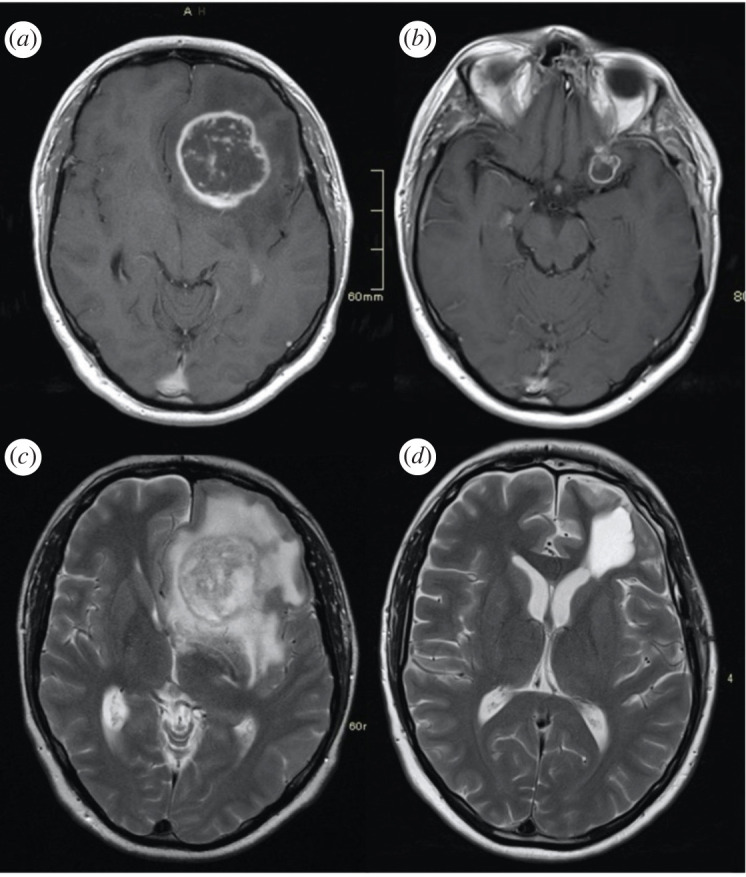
Representative images for a patient with remote recurrence of GBM in the contralateral hemisphere. T1-weighted post-gadolinium images (*a*,*b*) and T2-weighted images (*c*,*d*). (*a*) Pre-operative diagnostic imaging demonstrating a left frontal rim enhancing GBM with a significant volume of surrounding T2 hyperintensity. (*b*) Three months post-resection, post-adjuvant chemoradiotherapy (60 Gy in 30 fractions with concurrent temozolomide) baseline study.
